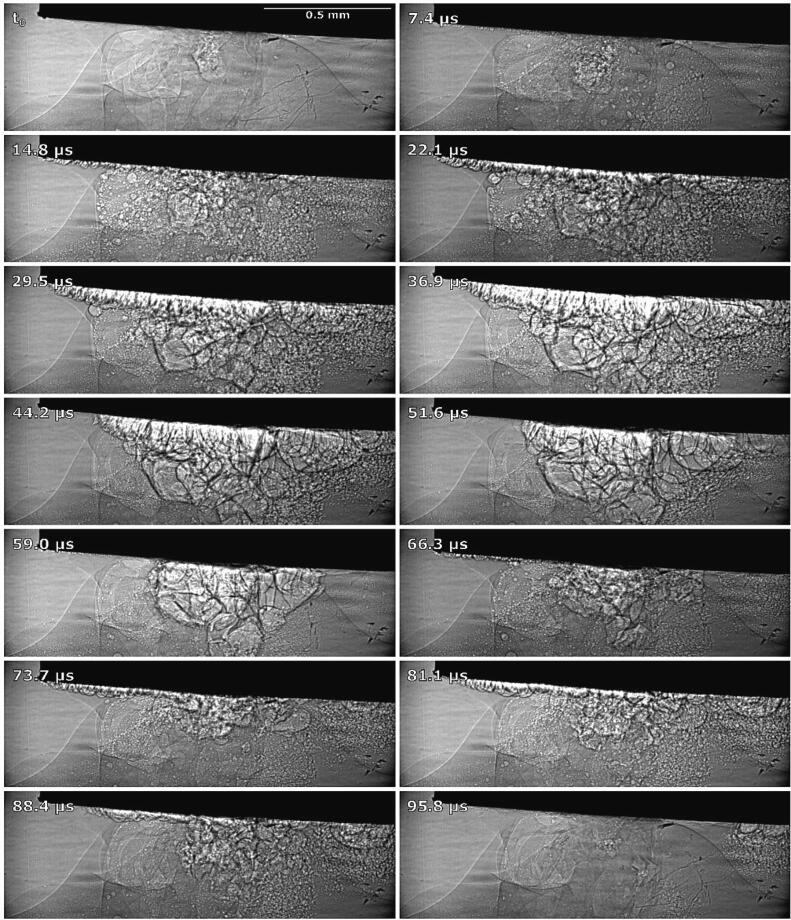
Synchrotron observations of emulsion stream formation: creation of coarser emulsion, cavitation bubble growth and dispersion of larger oil drops into finer emulsion during a 100 µs interval or 2 “oscillations” of the ultrasonic horn.
